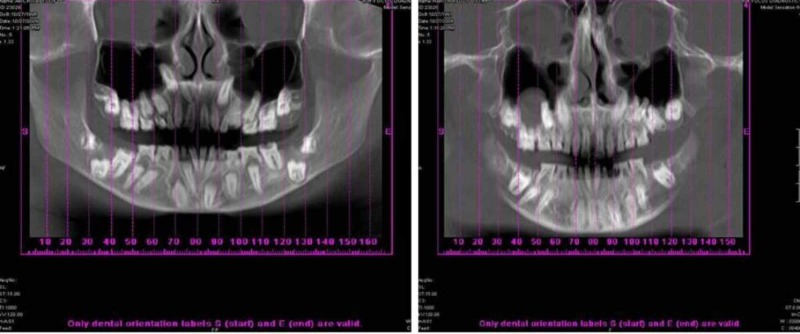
CT of maxilla and mandible of female and male patient
